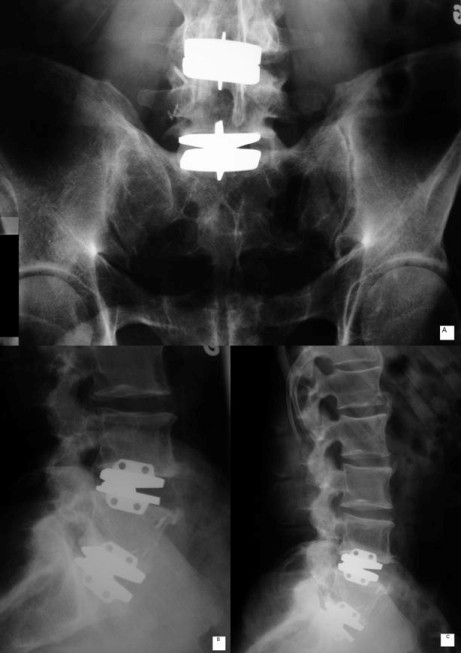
Metal-on-metal disc prosthesis at L4-L5 and L5-S1. a) antero-posterior radiograph, b) and c) lateral view.
